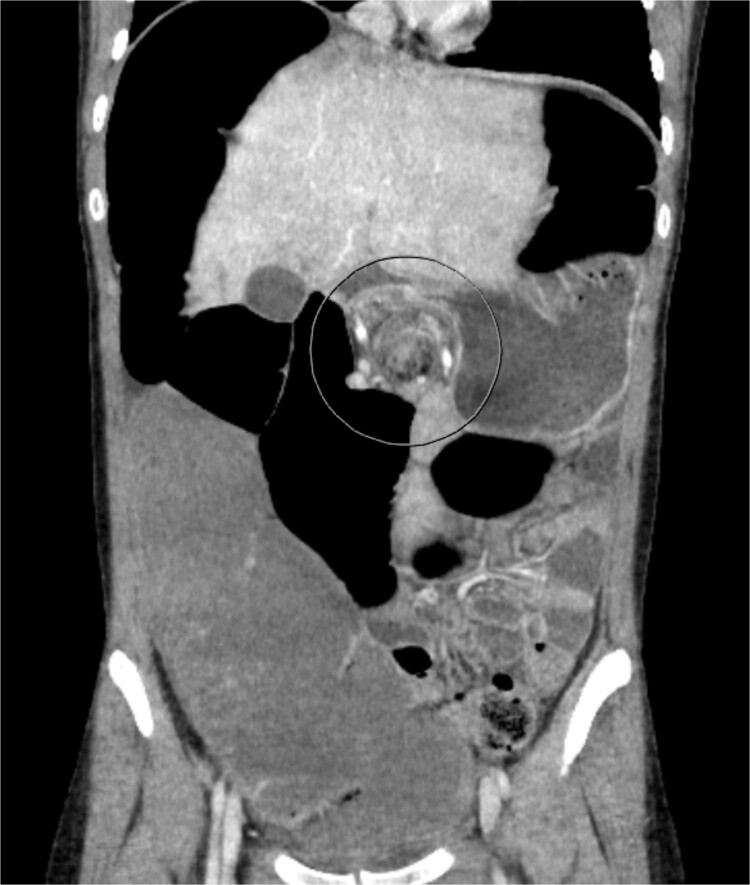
CT of the abdomen performed on Day 1 of admission. Shows colonic distension with volvulus at the splenic flexure.
